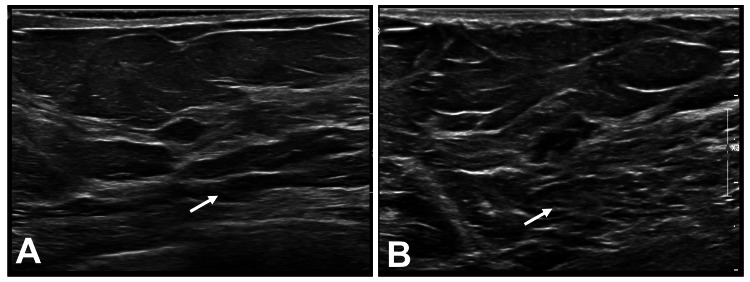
Ultrasound of the patient's left and right breasts Ultrasound of the patient's left (A) and right (B) breasts shows bilateral BI-RADS category 4 irregular, indistinct, hypoechoic masses measuring 6 mm and 30 mm in the left breast and 11 mm and 80 mm in the right breast, respectively (white arrows). BI-RADS: Breast Imaging-Reporting and Data System
